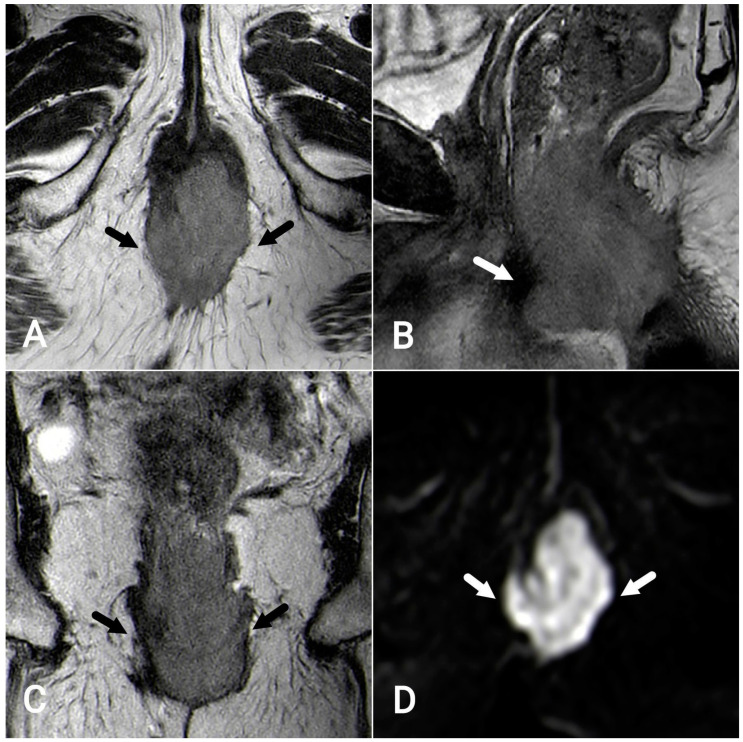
Squamous-cell carcinoma of the anal canal. Axial (A), sagittal (B), and coronal (C) T2-weighted MR images: heterogeneous lesion measuring 6.5 × 5.5 × 4 cm invading the left ischioanal fossa, internal and external sphincter muscles, and puborectalis muscle bilaterally. The tumor is characterized by restricted diffusion on diffusion-weighted imaging (D, white arrows).
